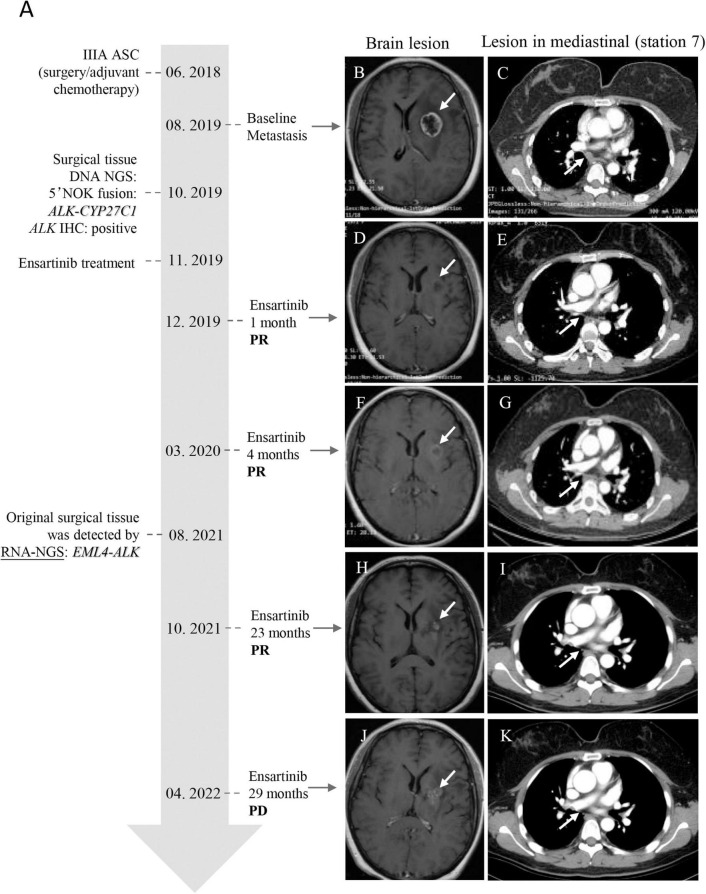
Timeline of the 5′ NOK *ALK* fusion clinical diagnosis and treatment and MRI/X-ray computed tomography of lesions of the brain and mediastinal lymph node (station 7) of the patient. (A) Timeline of patient clinical diagnosis and treatment. (B,C) Baseline: brain lesion (24*21 mm) and lymphadenopathy in the mediastinum (station 7) (short diameter, 10 mm). (D,E) One month after ensartinib treatment: brain lesion reduction (18*14 mm) and the lesion in the mediastinum (station 7) was reduced (short diameter, 4 mm). (F,G) Four months after ensartinib treatment: brain lesion reduction (16*15 mm) and the lesion in the mediastinum (station 7) disappeared. (H,I) Twenty-three months after ensartinib treatment: the brain lesion shrank again (15.8*13.8 mm), and there was no lesion in the mediastinal region (station 7). (J,K) Twenty-nine months after ensartinib treatment: the brain lesion progressed (28*21 mm), but the mediastinal region (station 7) was still responding. The white arrow indicates the lesion. ASC: adenosquamous carcinoma; PR: partial response; PD: progressive disease.
